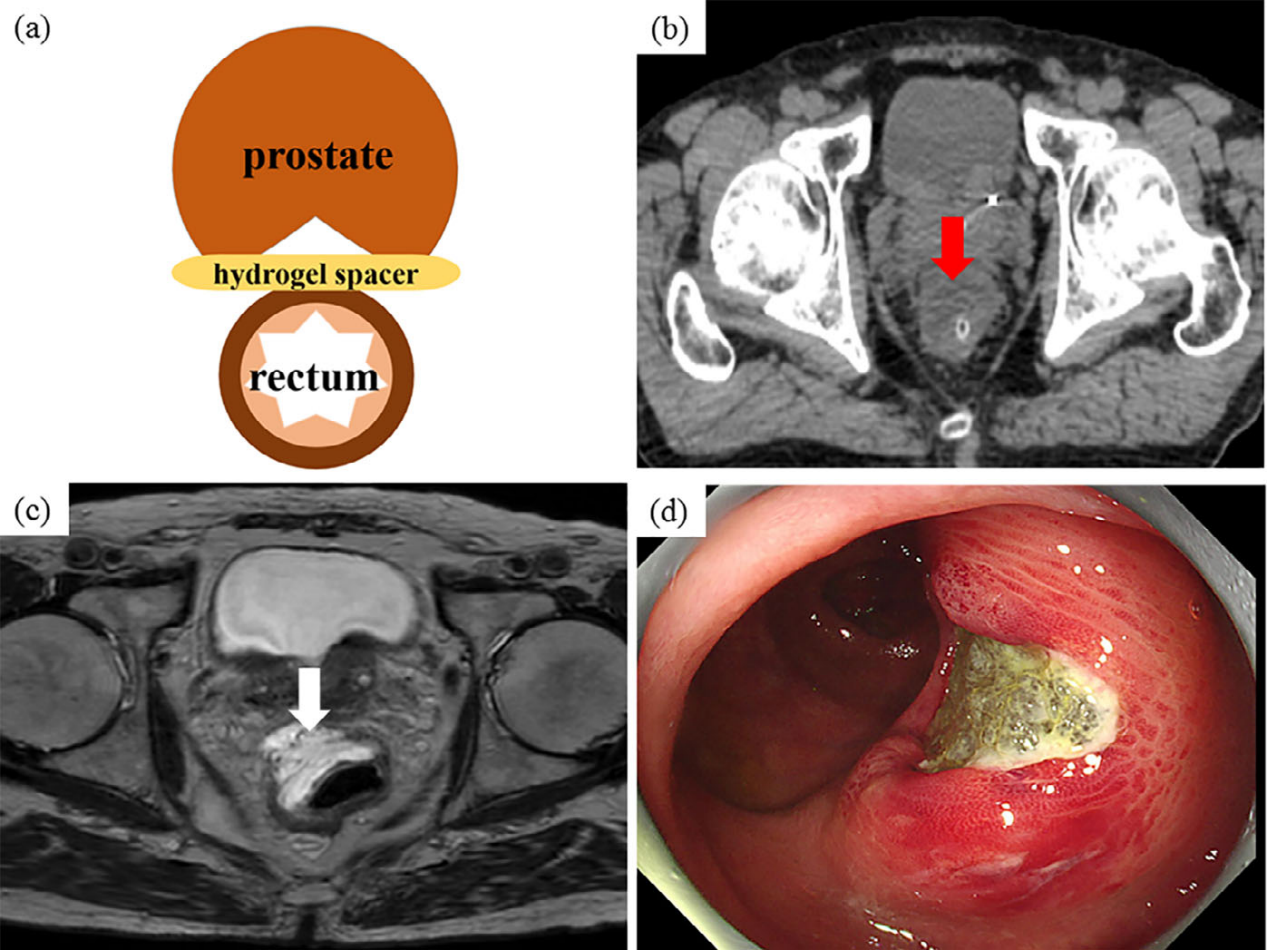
The hydrogel spacer is inserted transperineally between the anterior rectal wall and prostate fascia (a). Computed tomography image (b) of the abdomen shows a low‐density area that extends from the prostate to the rectal wall (red arrow). Axial T2‐weighted (c) and sagittal T2‐weighted magnetic resonance imaging images of the abdomen show the hydrogel spacer in the area between the anterior rectal wall and prostate as well as within the rectal wall (white arrow). An image obtained during colonoscopy (d) shows an approximately 2 cm ulcer in the rectum with bleeding around the hydrogel spacer.
